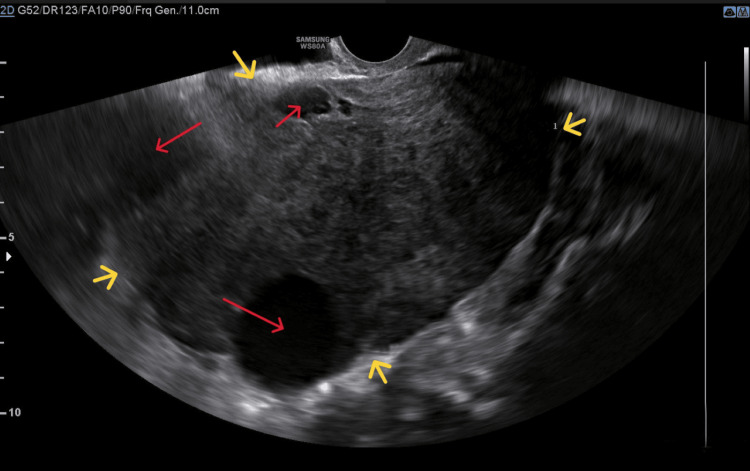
Transvaginal ultrasound imaging of the ovarian thecoma A well-defined pelvic mass (yellow arrows) is visible, primarily solid with some cystic elements (red arrows)
